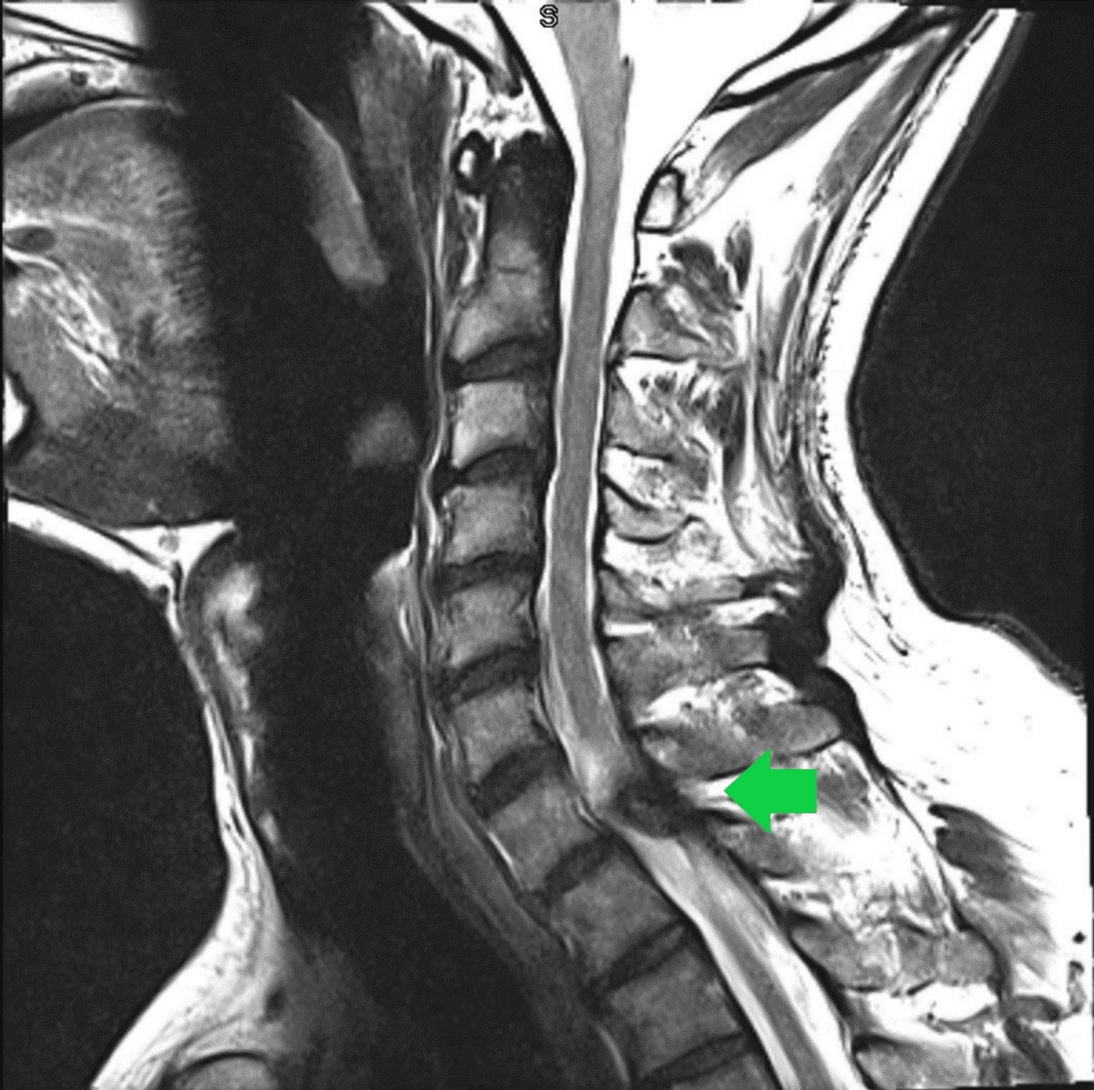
Sagittal MRI demonstrating the cyst and its relationship with the spinal cord.
